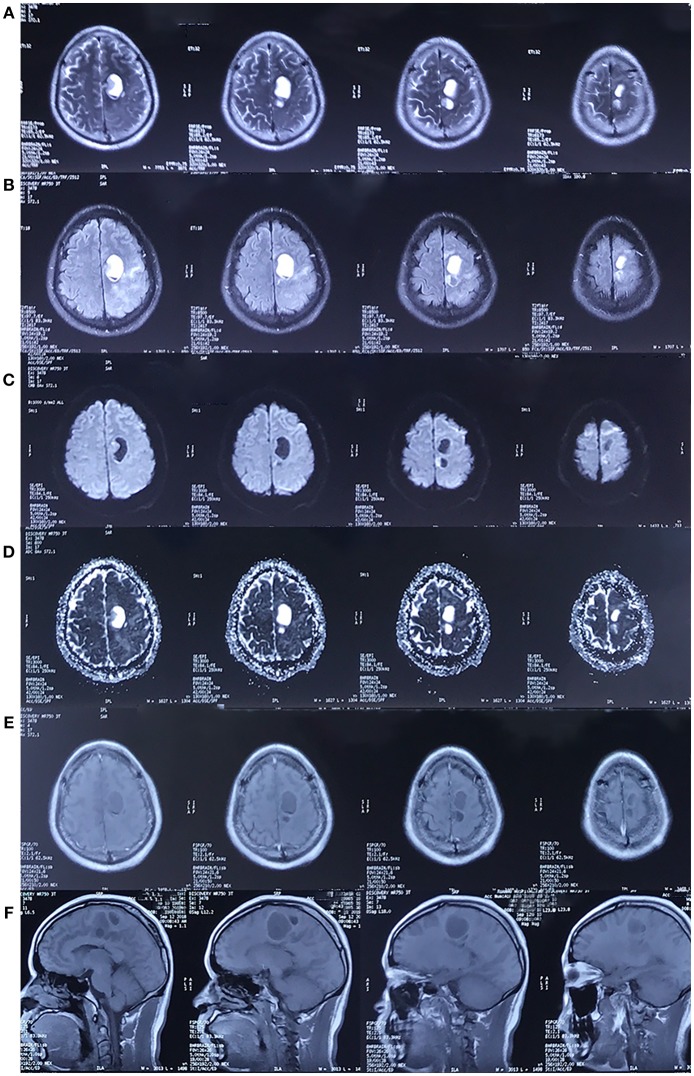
MRI in September 2018. The cystic lesion in left frontal lobe was enlarged. The lesion showed the same characteristics as the MRI scan performed in 2017. [(A–F) Represents T2-weighted image, FLAIR, DWI, ADC, axial and sagittal post-contrast T1-weighted image, respectively. The pre-contrast T1-weighted image was unavailable].
